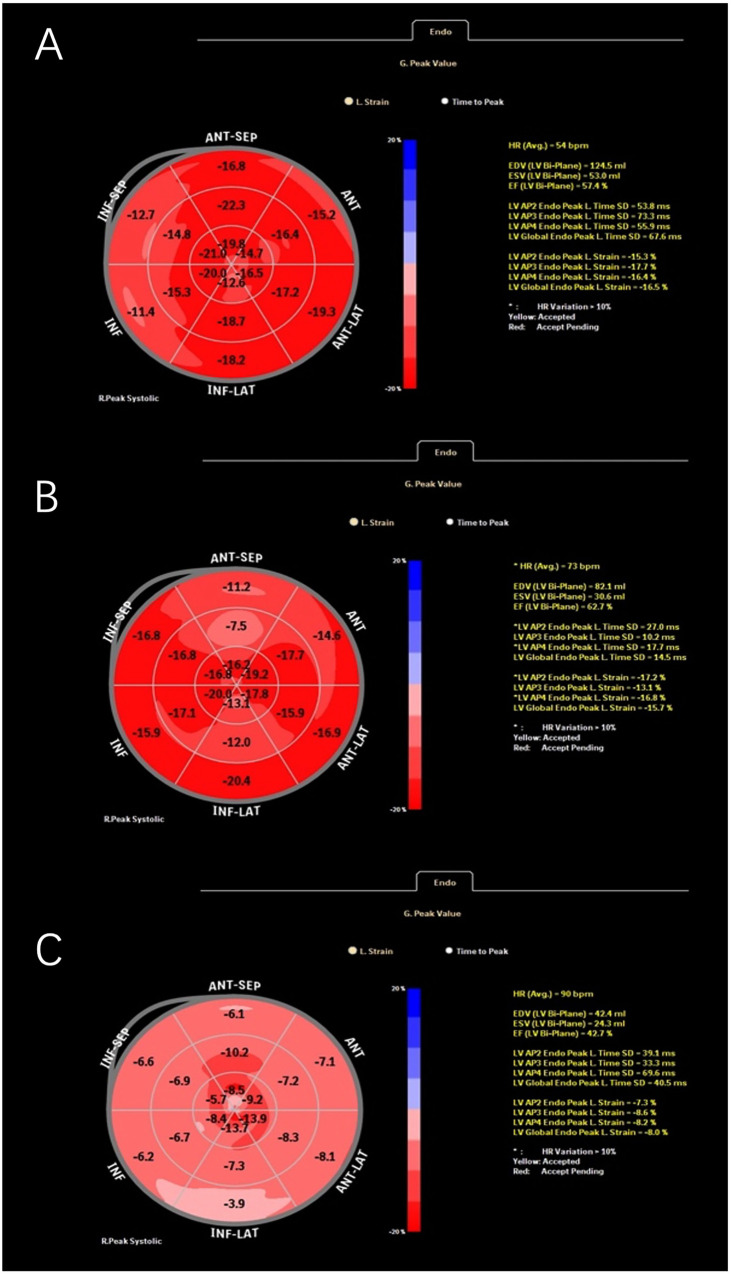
Representative global longitudinal strain (GLS) curves obtained from speckle-tracking echocardiography in different patients: ventricular premature patient 1 (A), ventricular premature patient 2 (B), and a patient with myocarditis (C).
